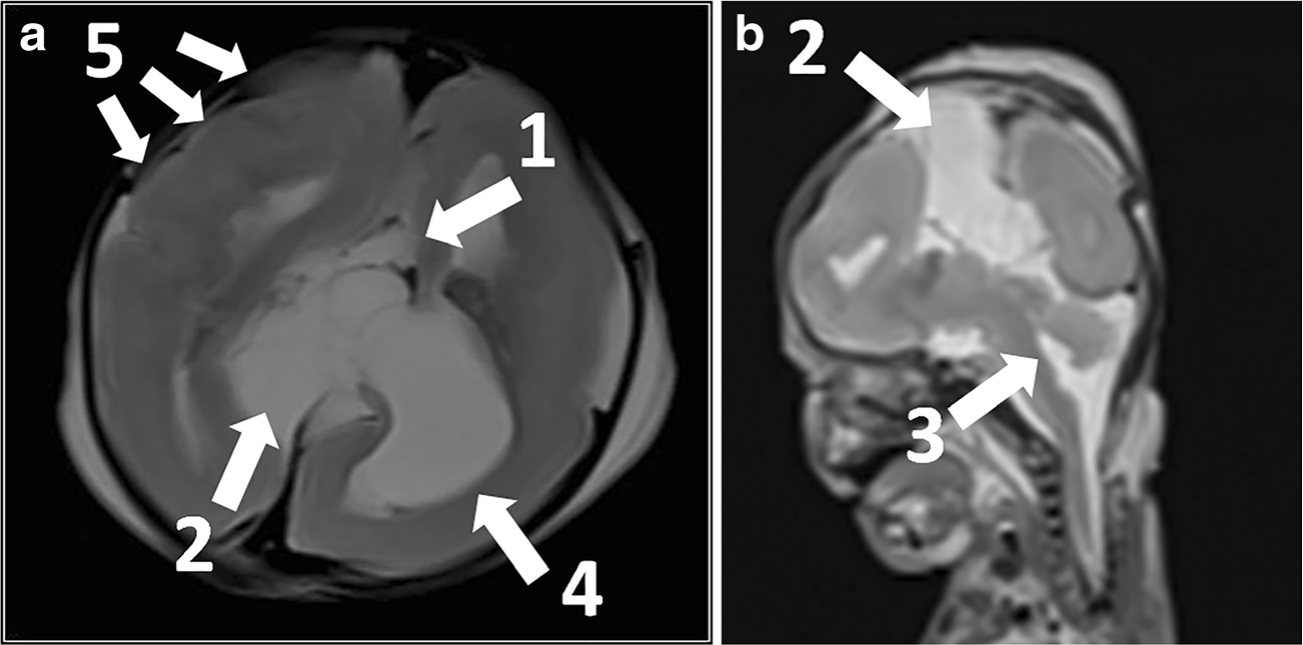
a, b Example of abnormalities of the central nervous system diagnosed at PMMRI in a female foetus. This pregnancy was terminated at 23 weeks of gestation because of corpus callosum agenesis (1), an interhemispheric cyst (2) and fossa posterior anomalies on prenatal 2nd trimester ultrasound, which were confirmed by PMMRI and/or conventional autopsy. A non-cystic dilatation of the fourth ventricle (3) was found on PMMRI along with the additional findings of a left choroid plexus cyst (4) and polymicrogyria (5). Furthermore, autopsy diagnosed a choroid plexus papilloma in the left lateral ventricle, but the additional finding of polymicrogyria (5) on PMMRI revealed Aicardi syndrome as the most likely diagnosis. a Axial. b Sagittal
